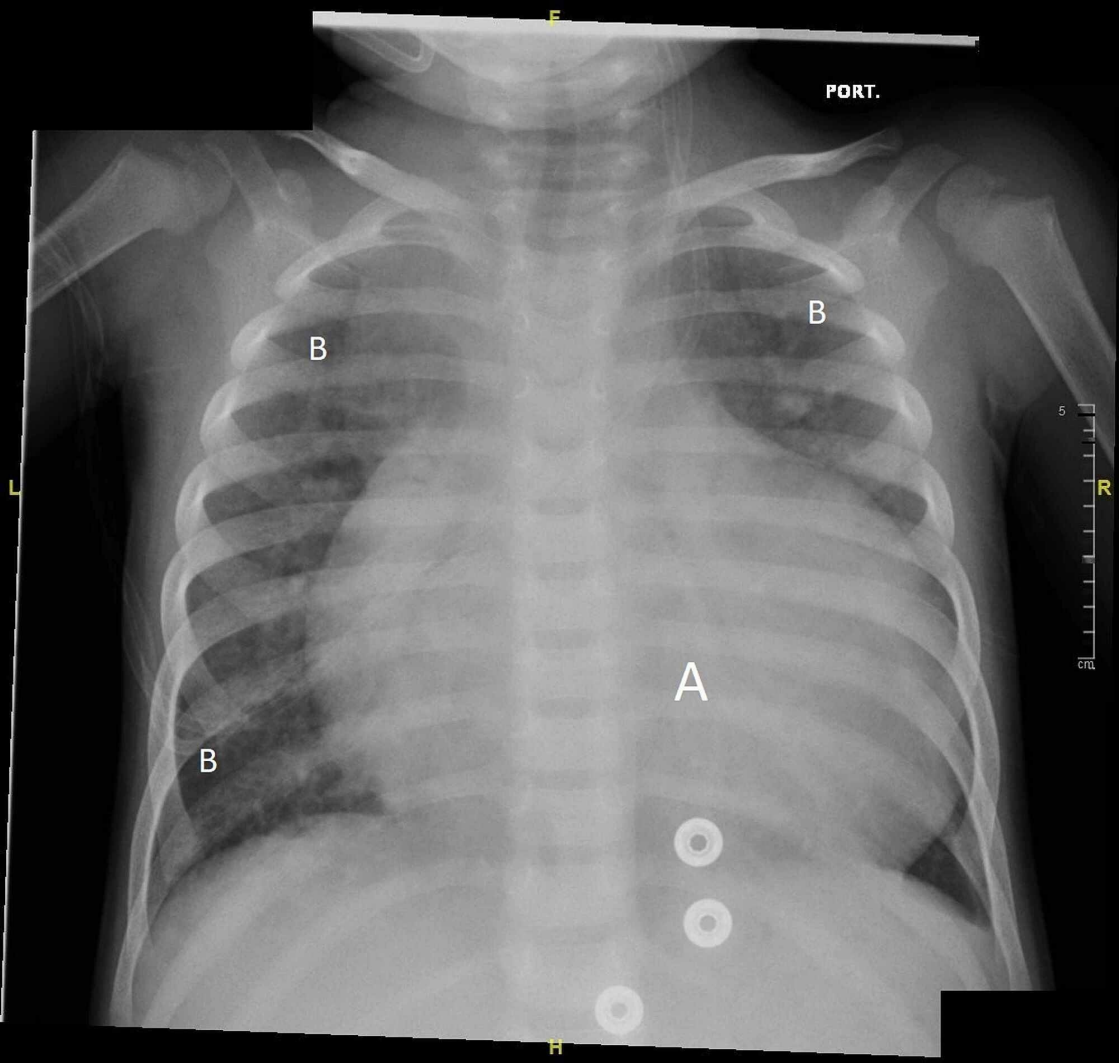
Second CXR on the fifth day of admission. A: cardiomegaly; B: improvement of chest consolidation CXR, chest X-ray
